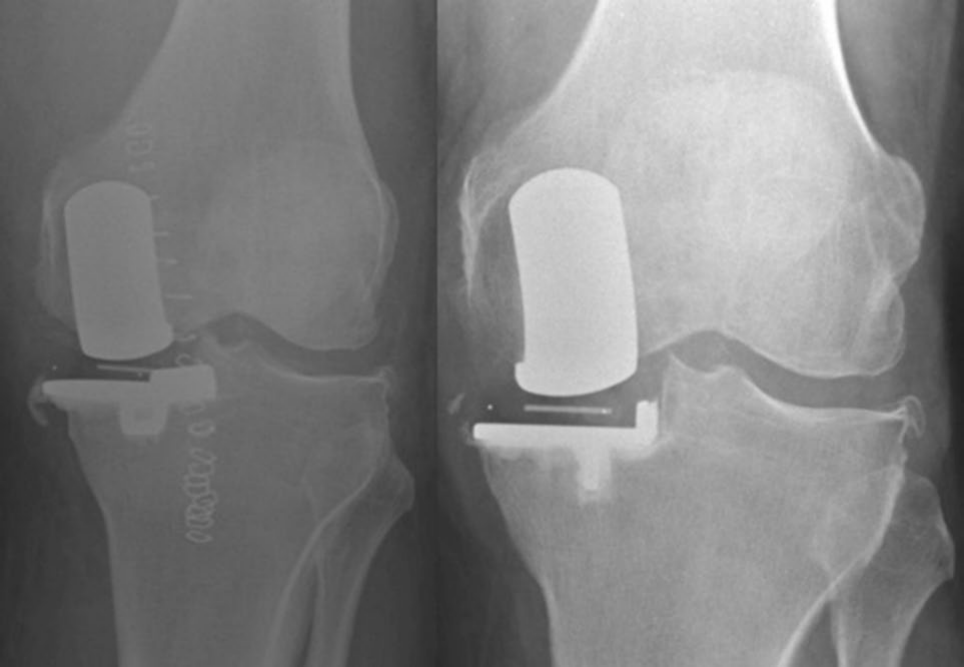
Radiograph on the left demonstrating what appears to be significant medial overhang and malalignment of the tibial component. A subsequent aligned radiograph of the same knee demonstrating a perfectly aligned tibial component. Note the presence of excess cement in and around the joint
